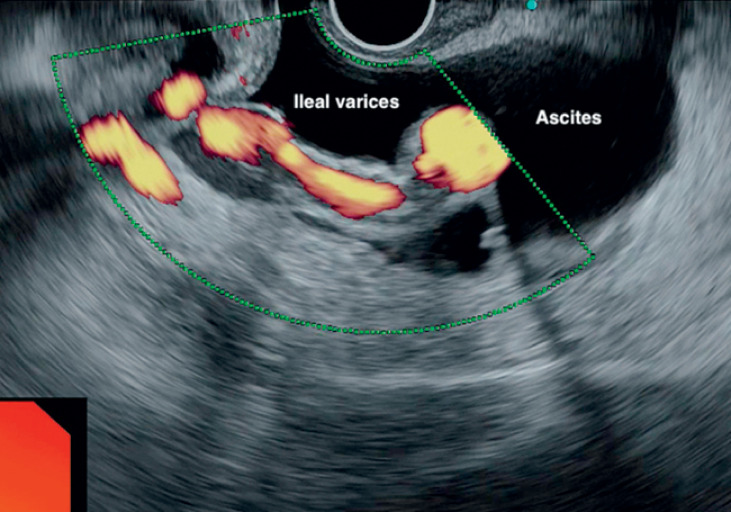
Rectal endoscopic ultrasound image showing significant ascites with ileal varices floating in the fluid.
